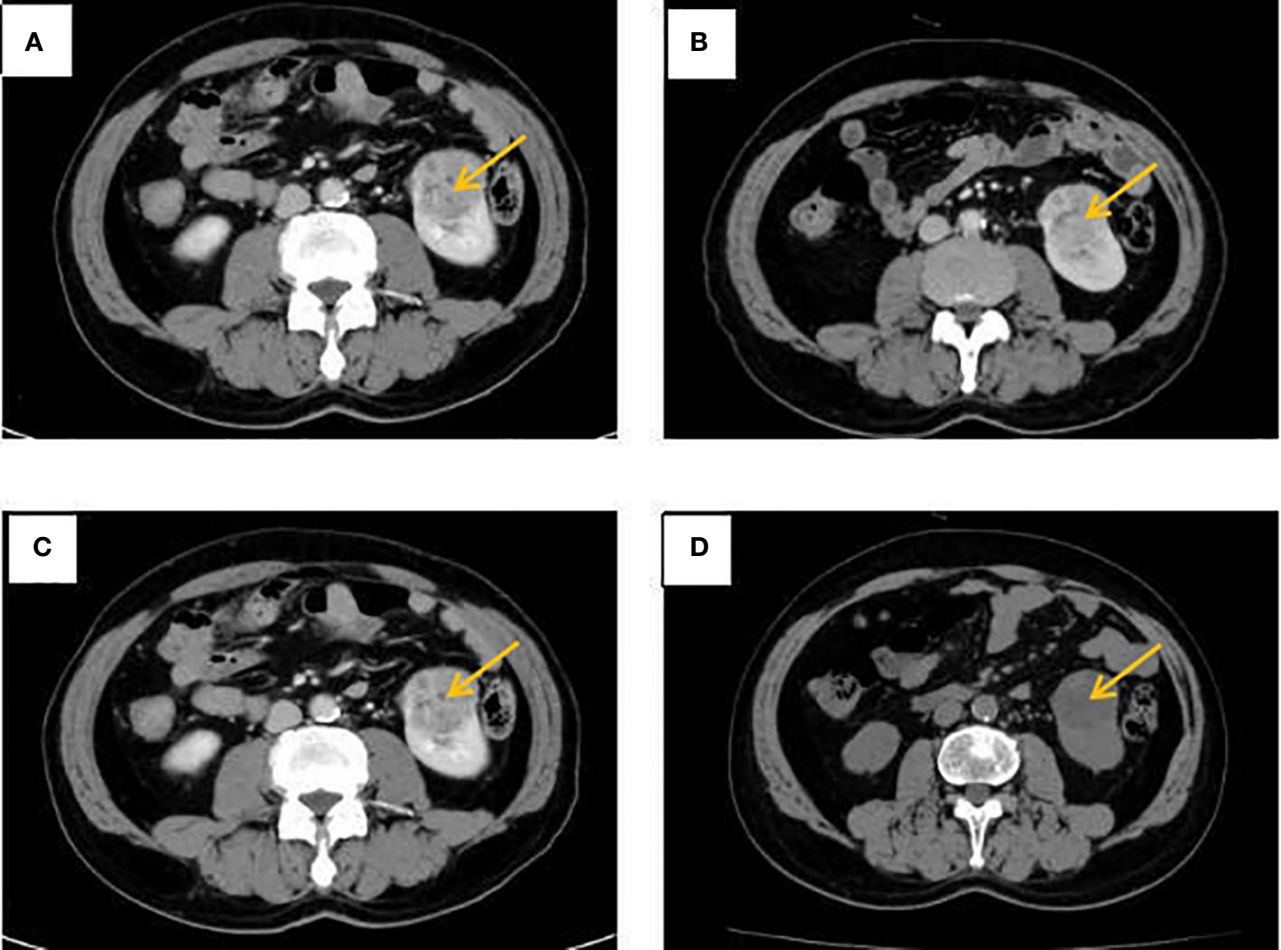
Abdominal CT at different times. (A) The left kidney lesion before treatment. (B) CT revealed a stable disease after 2 cycles of albumin-bound paclitaxel and cisplatin in combination with sintilimab. (C) CT revealed a stable disease after 6 cycles of albumin-bound paclitaxel and cisplatin in combination with sintilimab. (D) No significant change in left kidney lesion after sequential 2 cycles of sintilimab immune monotherapy maintenance treatment.
